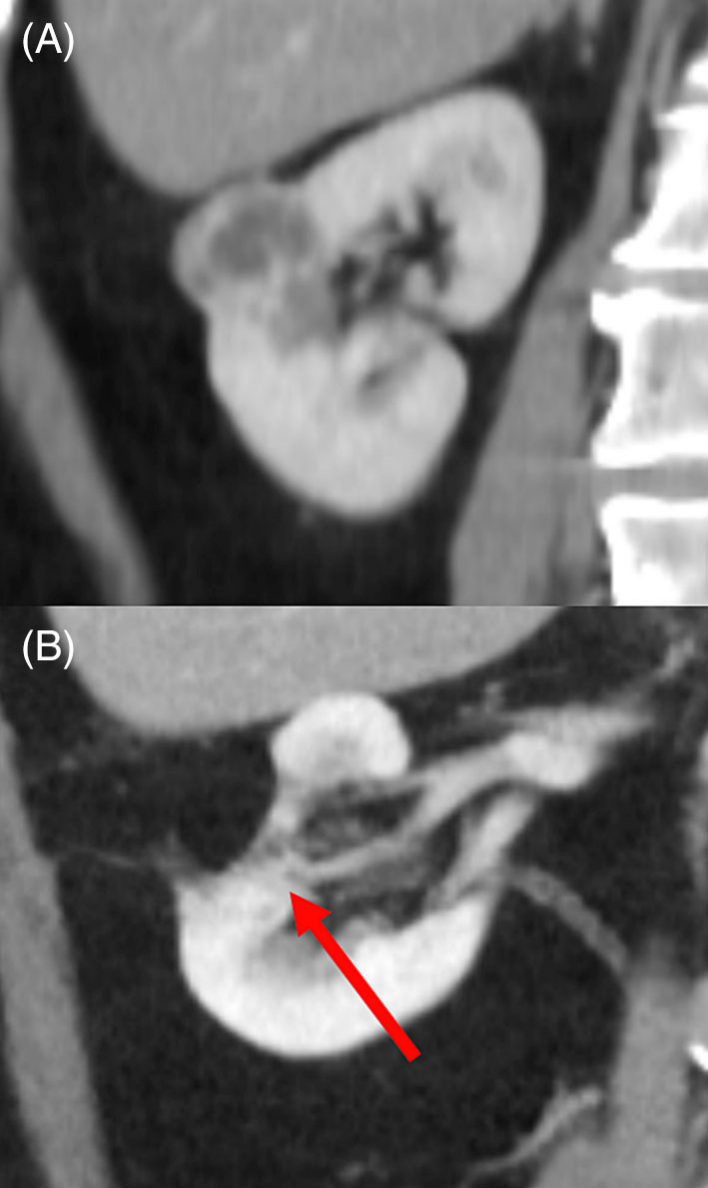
Computed tomography images of (A) pre‐ and (B) post‐partial nephrectomy, when a pre‐gerotal fat patch has been used as a haemostatic bolster.
